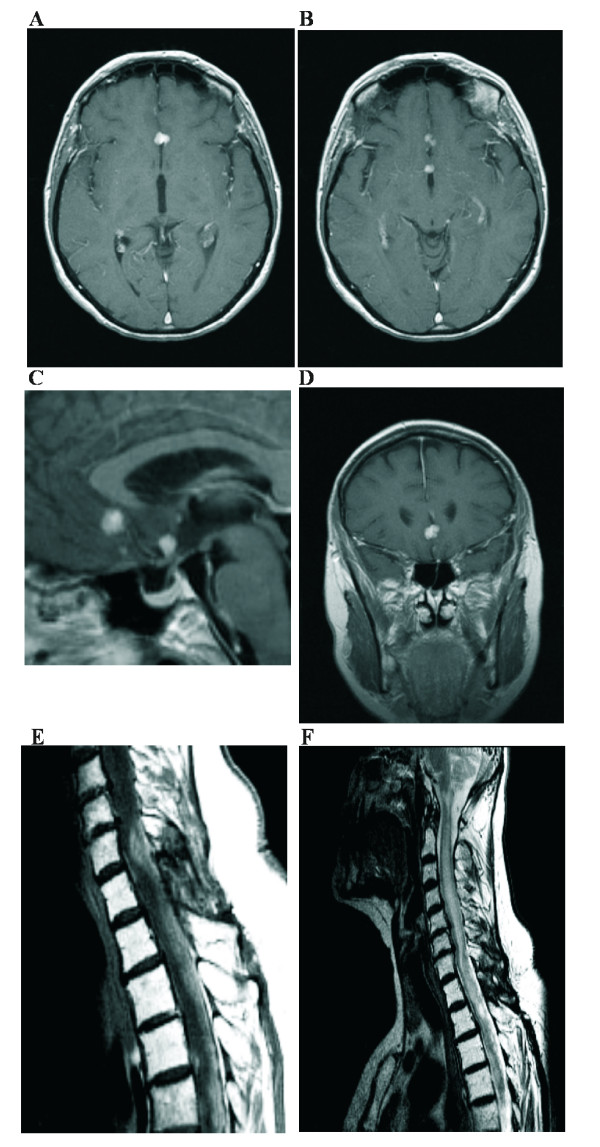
December 2004: Contrast-enhanced axial (A, B), sagittal (C), and coronal (D) T1-weighted MR-images of the brain and sagittal T1- and T2-weighted MR-images (E, F) of the spine showing multiple intracranial metastatic nodules and extensive spinal involvement.
